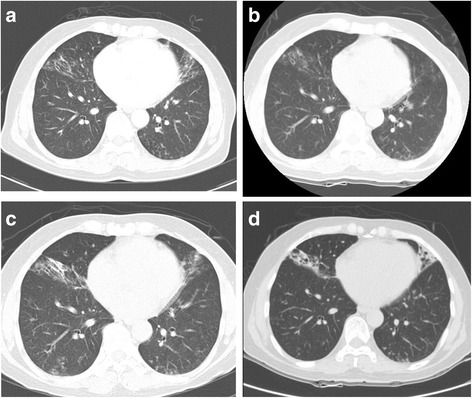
a Chest CT scan of a patient with symptomatic *Mycobacterium intracellulare* lung disease before initiation of treatment. The scan shows bronchiectasis, nodules, and reticular densities in the right middle lobe and lingular segment. Treatment started 4 weeks after checking this CT scan. b Chest CT scan at 6 months after the initiation of treatment showing substantial improvement. c Chest CT scan at 12 months after the completion of 18-month treatment for *M. intracellulare* lung disease. Radiographic lesions and symptoms worsened; bloody sputum was also noted. Four weeks after this CT scan was taken, *M. abscessus* subspecies *abscessus,* instead of *M. intracellulare,* was isolated. d Chest CT scan at 6 months after initial isolation of *M. abscessus* subspecies *abscessus*. The patient’s symptoms continued to worsen and sputum persistently tested positive for *M. abscessus* subspecies *abscessus*
